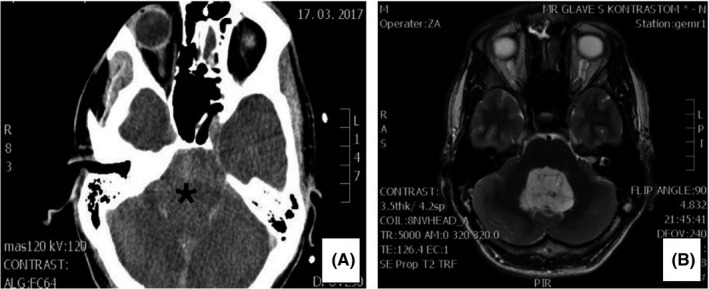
Imaging features of the RGNT. A, CT‐scan with contrast. A mildly hypodense mass lesion (asterisk) in the fourth ventricle measuring 30 × 40 × 40 mm. There was no enhancement after contrast administration. B, MRI, T2‐weighted image. Well‐demarcated, abundant tumor in the fourth ventricle with surrounding edema
